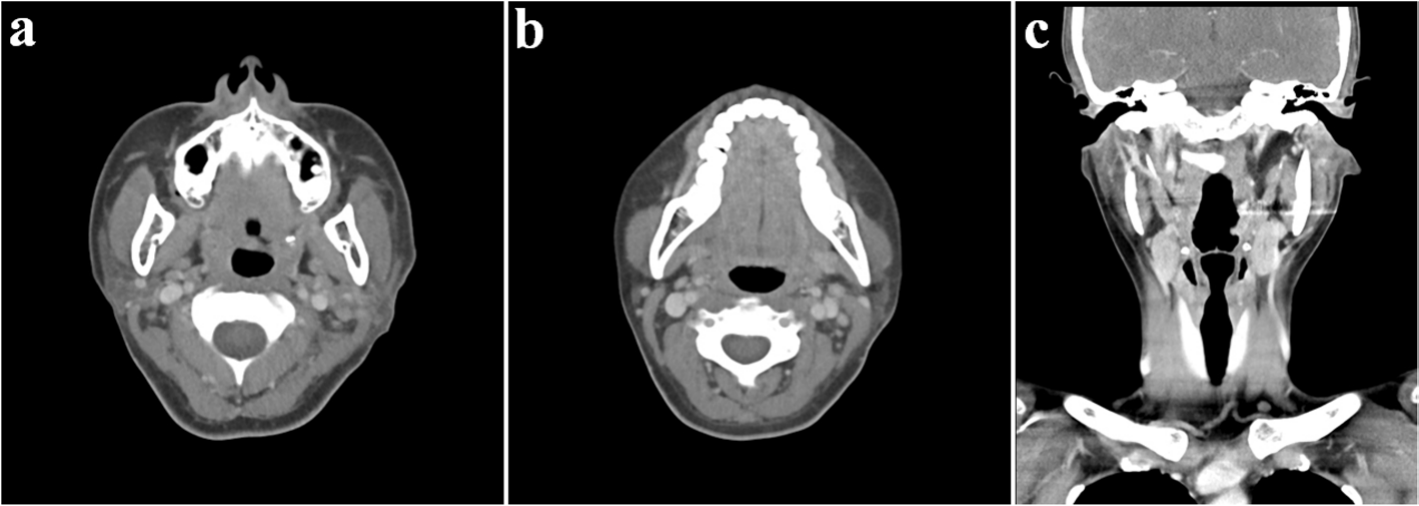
Postoperative CT images on day 8. a Axial view (parotid gland area). b Axial view (lymph node area, level II, III); decreased size of the lymph nodes. c Coronal view
